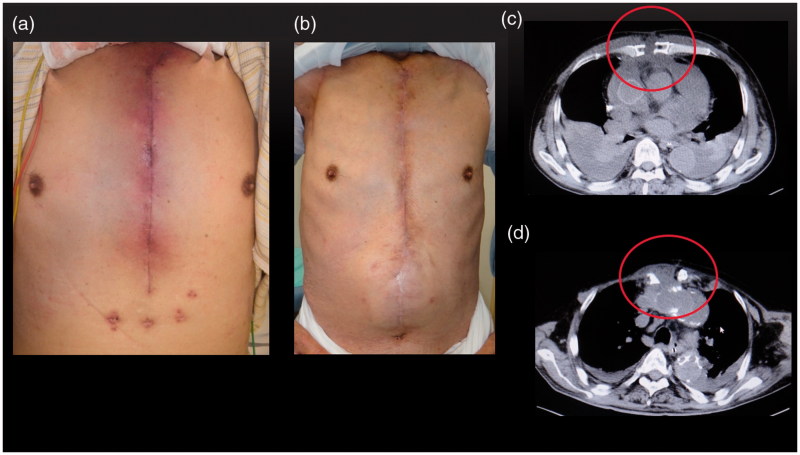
(a) Clinical observation: erythema at the precordial operation scar. (b) Six months after surgery: no recurrence. (c) Preoperative computed tomography: sternal ablation and liquid retention. (d) Postoperative computed tomography: dead space filled with a pectoralis major muscle flap.
